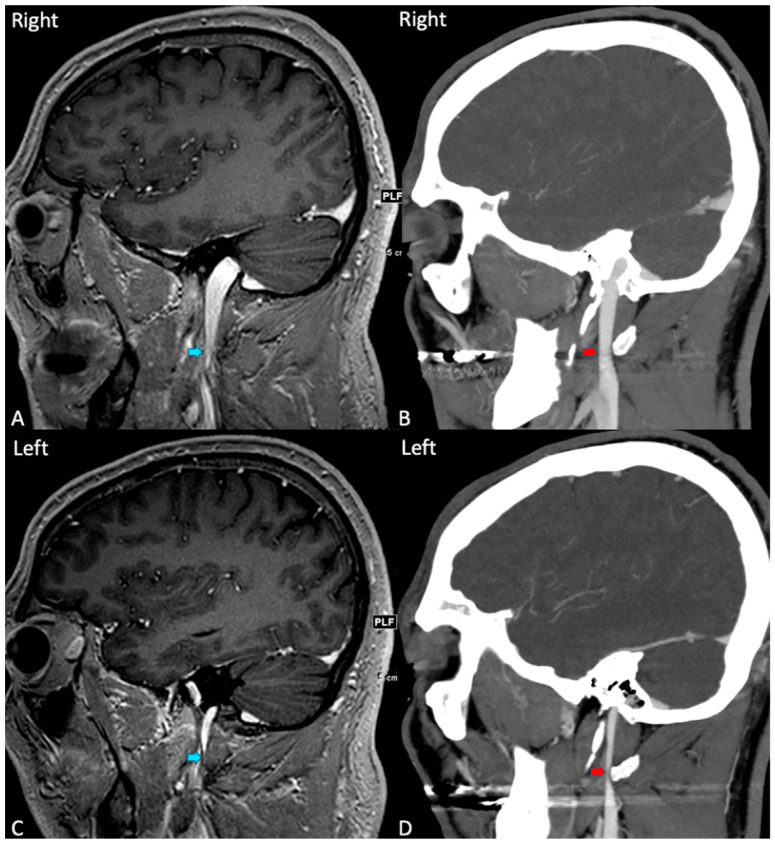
Apparent IJVS on MRI with vessel patency on CT venogram: Sagittal MRI brain with contrast ((A) on the right side and (C) on the left side) showing tapering and apparent stenosis of both internal jugular veins (blue arrows). Sagittal CT venogram with contrast ((B) on the right side and D on the left side) of the same patient showing normal size of the right internal jugular vein (red arrow, (B)) and moderate narrowing of the left jugular vein caused by the lateral mass of C1 (red arrow, (D)).
